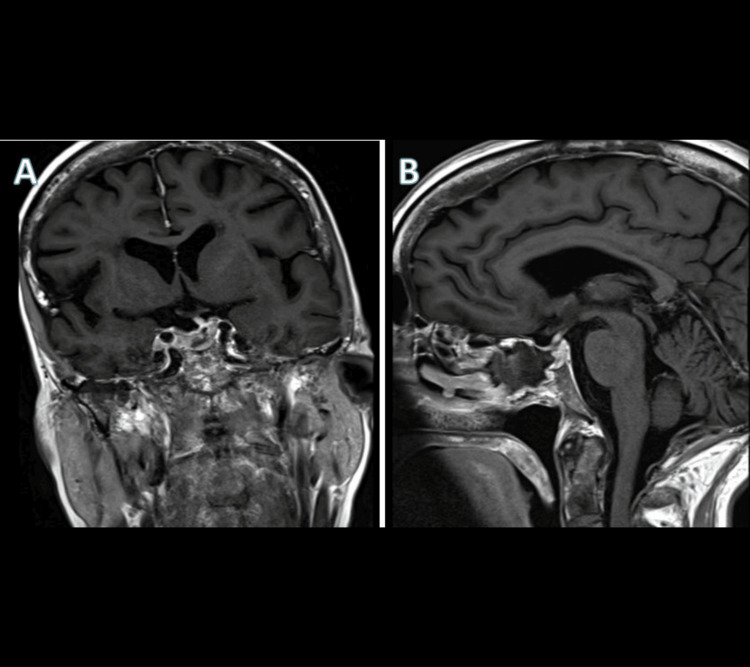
Postoperative T1 MRI with contrast (A) Coronal view. (B) Sagittal view MRI: magnetic resonance imaging
